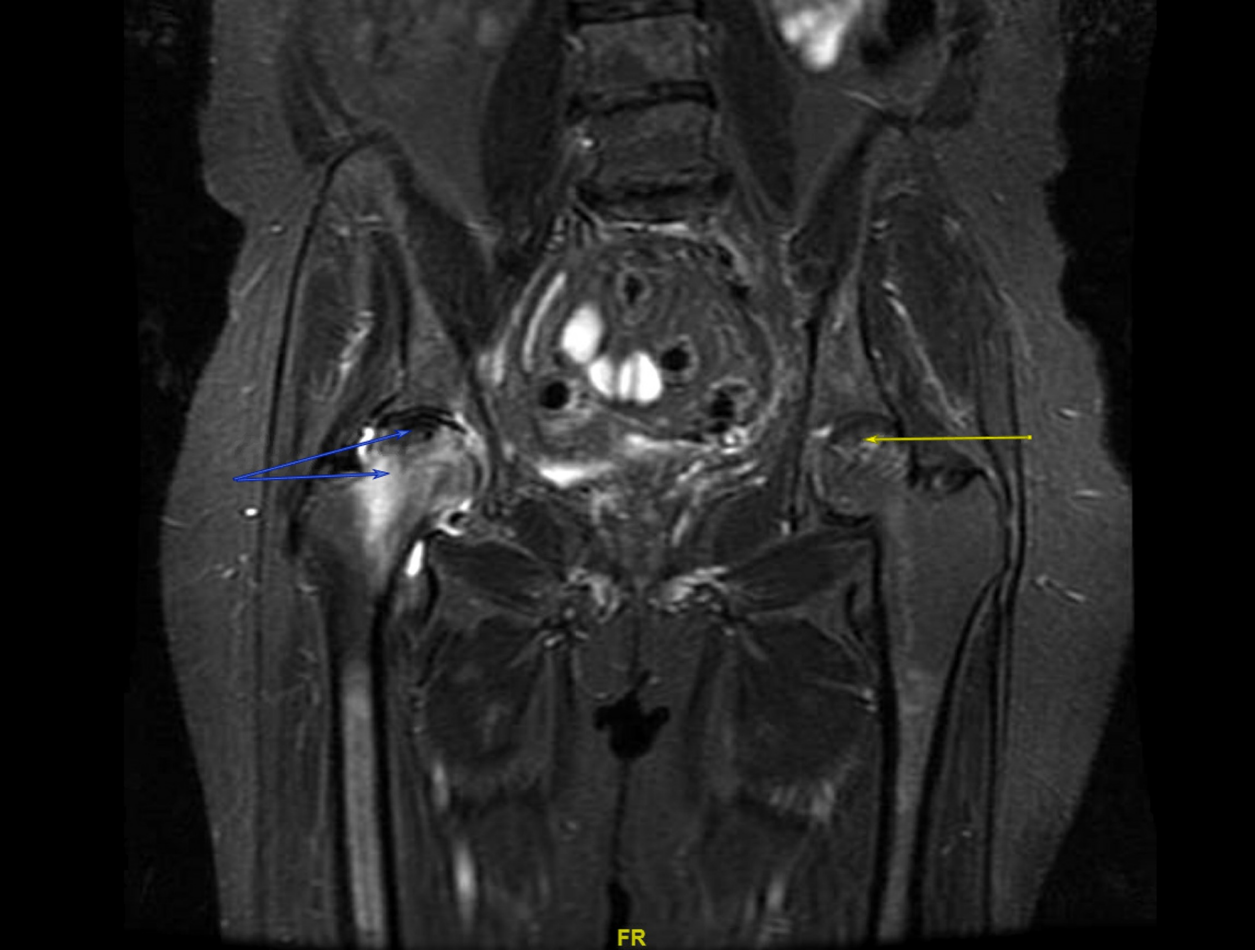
MRI of the pelvis and both hips without contrast showing subacute avascular necrosis of the left hip (yellow arrow), and acute, subacute, and chronic avascular necrosis of the right hip (blue arrows).
